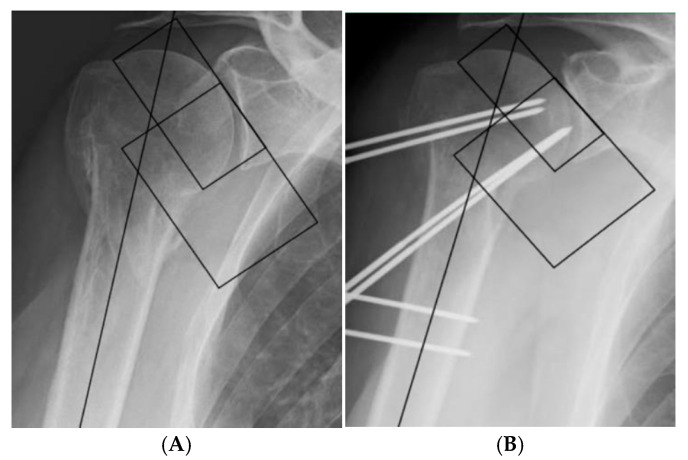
(A) Preoperative radiograph: the shoulder joint is positioned parallel to the body and perpendicular to the X-ray beam. The image is obtained with the C-arm rotated 45° posteriorly, with the beam directed through the center of the glenohumeral joint to optimize visualization of the surgical field and intraoperative anatomy. (B) Intraoperative radiograph after fixation with six fully threaded 2.5 mm pins to stabilize the proximal humerus.
